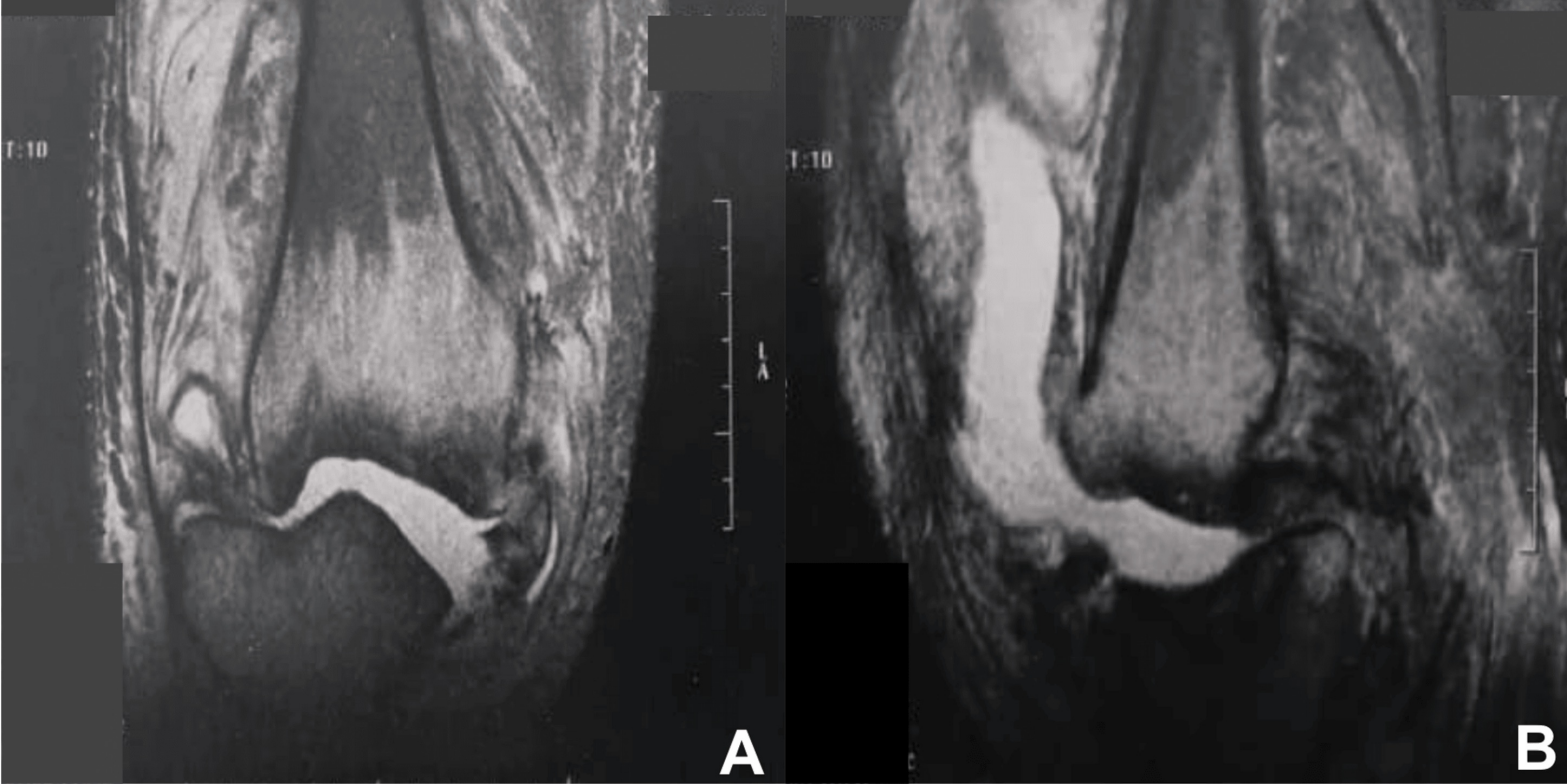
MRI of the right knee: (A) sagittal T2-weighted sequence and (B) sagittal T2-weighted STIR sequence MRI sequences of the right knee showing extensive destruction of the medial femoral condyle with osteolysis of the medial tibial plateau associated with periarticular bone remodeling. STIR: Short tau inversion recovery.
